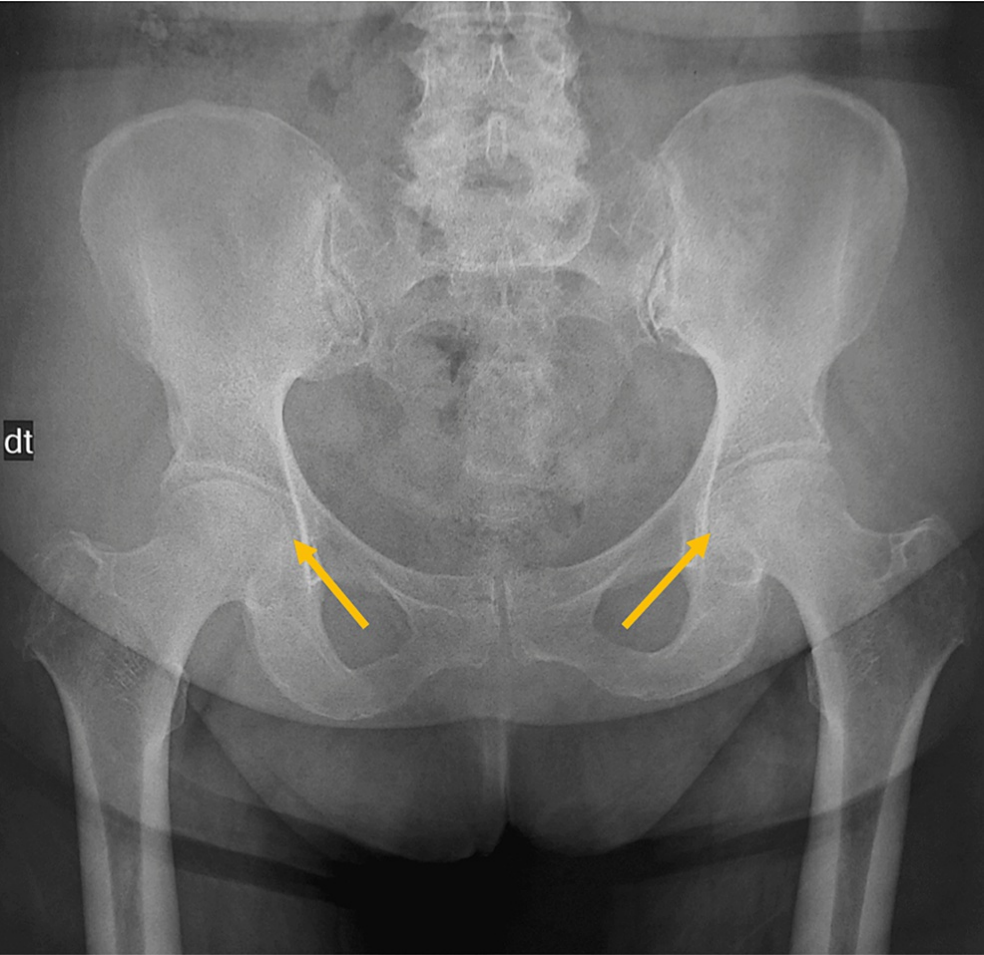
Standard radiography shows loss of sphericity of the femoral head.
